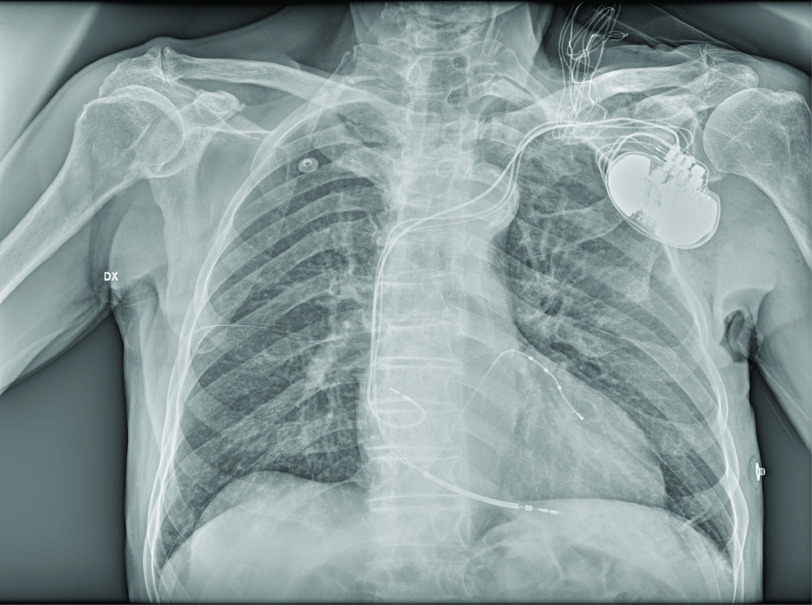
Bedside chest x-ray showing the cardiac resynchronization therapy defibrillator (Biotronik Rivacor 7 HF).
